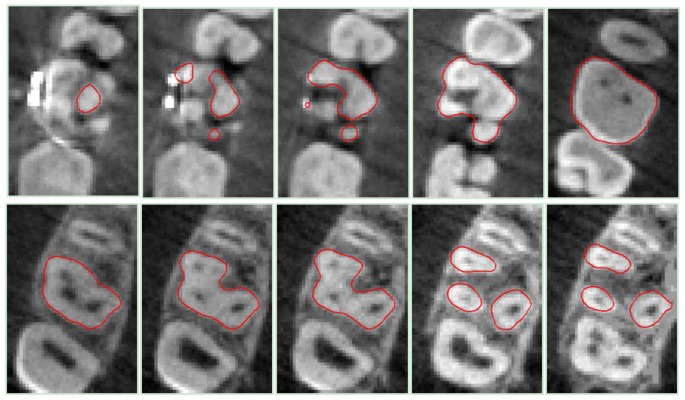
Results of the DRLSE method for tooth 16, obtained by processing some CBCT slice images.
